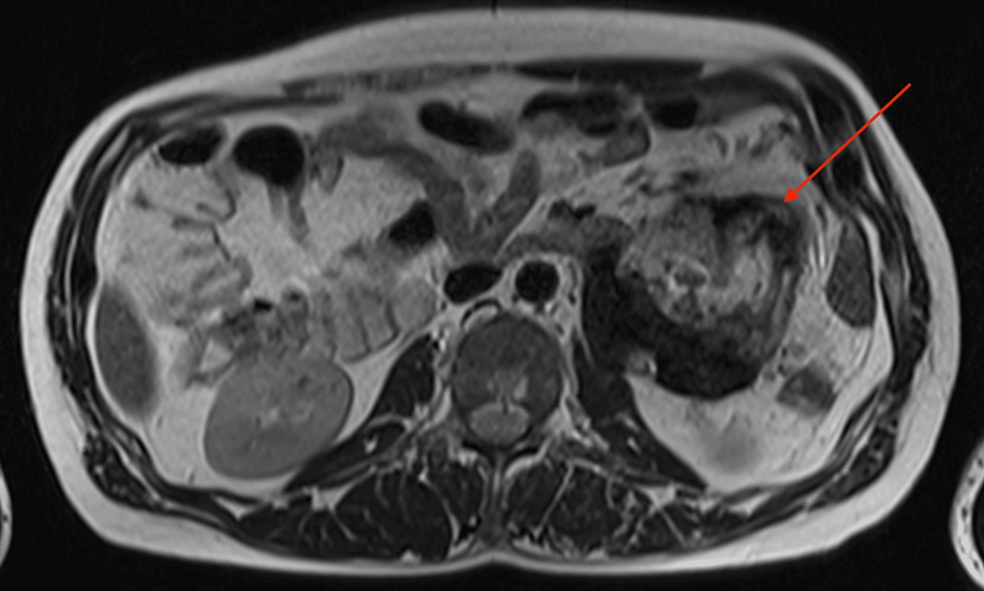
Axial MRI T2-weighted image MRI: magnetic resonance imaging
